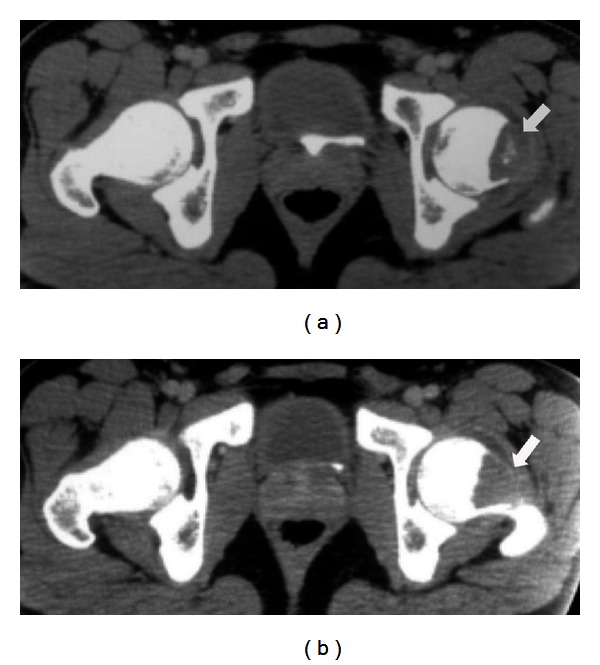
Axial CT scan of the pelvis showing (a) involvement of anterior aspect of neck of left femur with anterior cortical destruction and (b) the same lesion extending into the head of the left femur.
